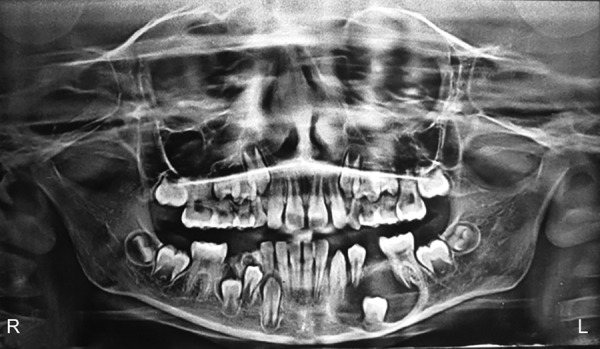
Orthopantomogram (OPG) showed a large radiolucent lesion with erupting 2nd premolar in left mandibular body region
